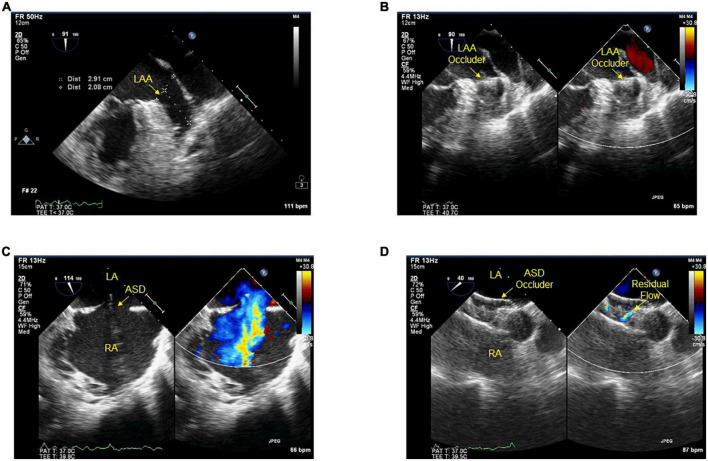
(A–D) Transesophageal echocardiography during procedures. Before (A) and after (B) the device implanted into the ostium of LAA. Before (C) and after (D) ASD closed with the device, residual flow ≤2 mm around the device after implantation. LAA, left atrial appendage; ASD, atrial septal defect; LA, left atrium; RA, right atrium.
